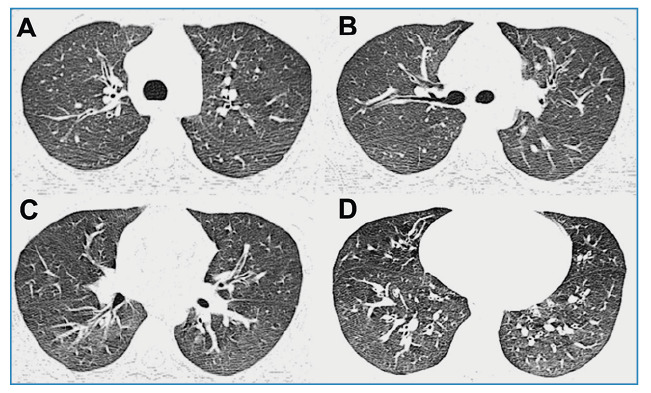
Axial CT images (lung window) showing diffuse thickening of the bronchial walls in a 17-year-old male with pertussis. CT: Computed tomography
